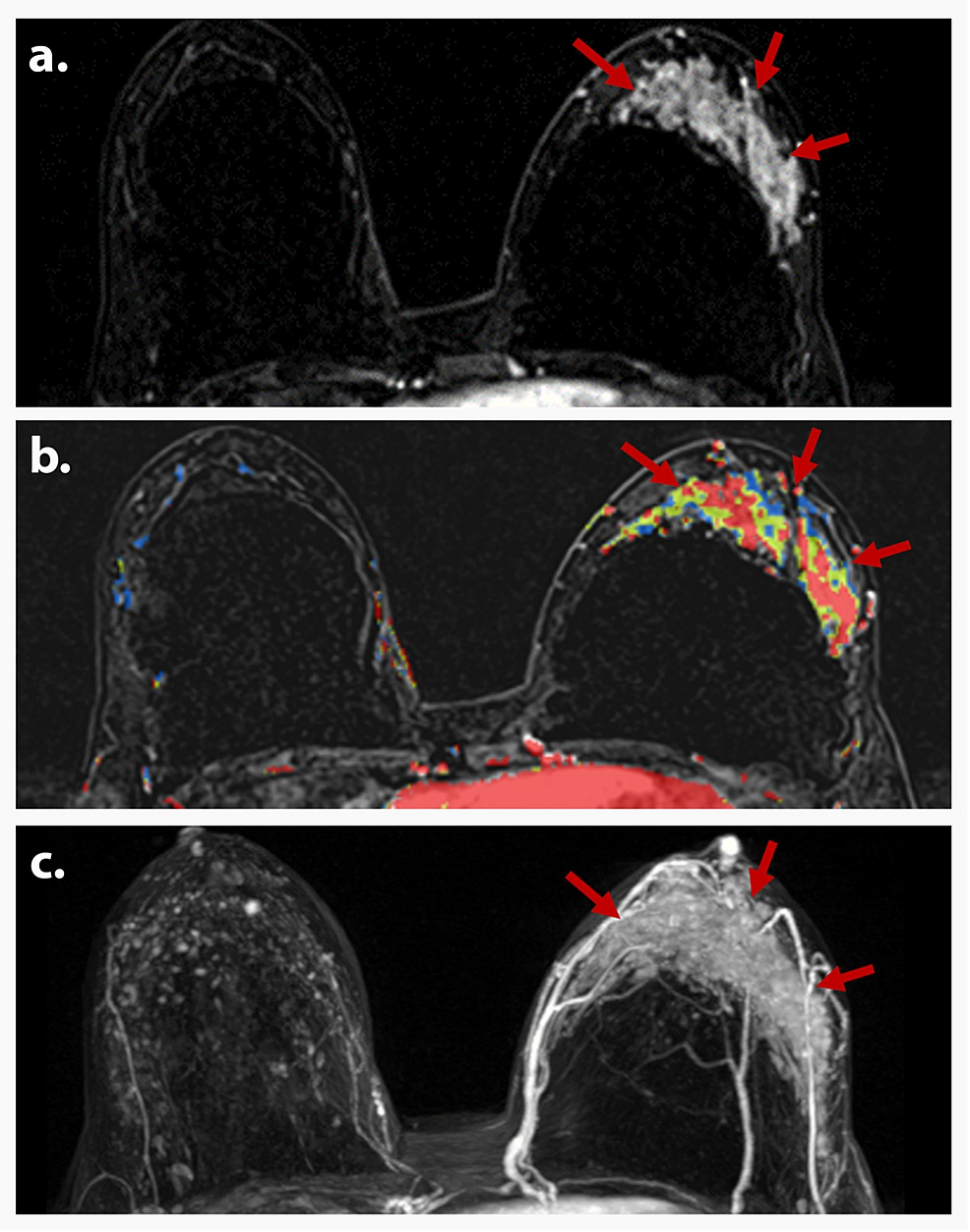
AJCC TNM stage 0 (TisN0M0) A 38-year-old premenopausal woman presented with a palpable left breast mass. The diagnostic mammogram was normal. Axial T1-weighted DCE (a), corresponding color-coded map (b), and MIP (c) magnetic resonance images show diffuse non-mass enhancement (red arrows) in the left breast with mixed kinetics. Biopsy depicted DCIS. Findings are consistent with AJCC TNM stage 0 (TisN0M0). AJCC: American Joint Committee on Cancer; TNM: tumor, node, and metastasis; DCE: dynamic contrast-enhanced; MIP: maximum intensity projection; DCIS: ductal carcinoma in situ.
